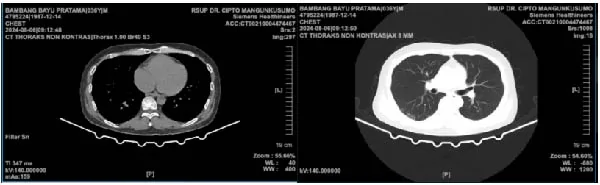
Chest CT scan results showed reticular opacities and surrounding ground‐glass opacities in both lungs.
